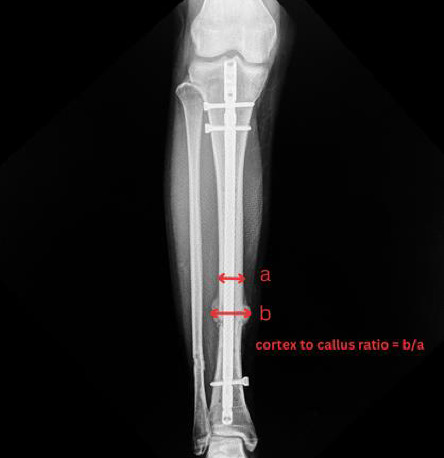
Radiographic measurement of the cortex-to-callus ratio. The cortical bone thickness (a) and total callus thickness (b) were measured at the fracture site. The cortex-to-callus ratio was calculated as b/a, representing the proportion of callus formation relative to the original cortex.
